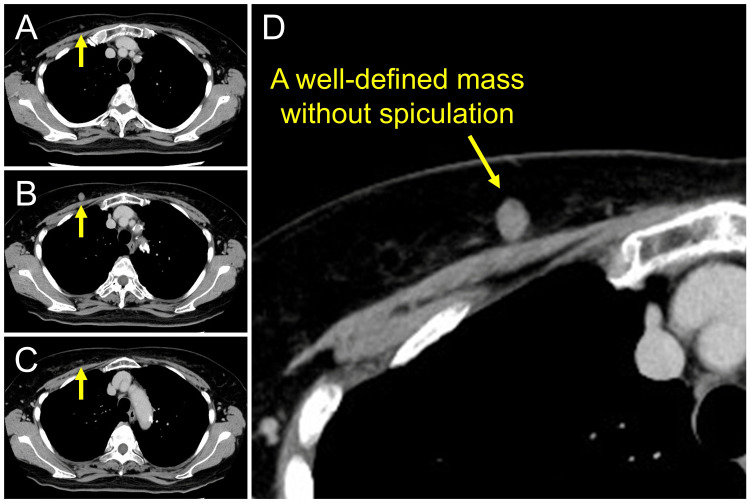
Whole-body computed tomography performed for evaluation of vascular access incidentally revealing a breast lesion Chest CT with 5-mm slice thickness revealed a well-defined mass in the right breast, showing a few malignant features such as spiculation. Serial images across three consecutive slices (A-C) and an enlarged view of the lesion (D) are shown. The yellow arrows in panels A-C indicate the breast mass. The lesion is clearly visible in panel B but only partially visible in panels A and C.
